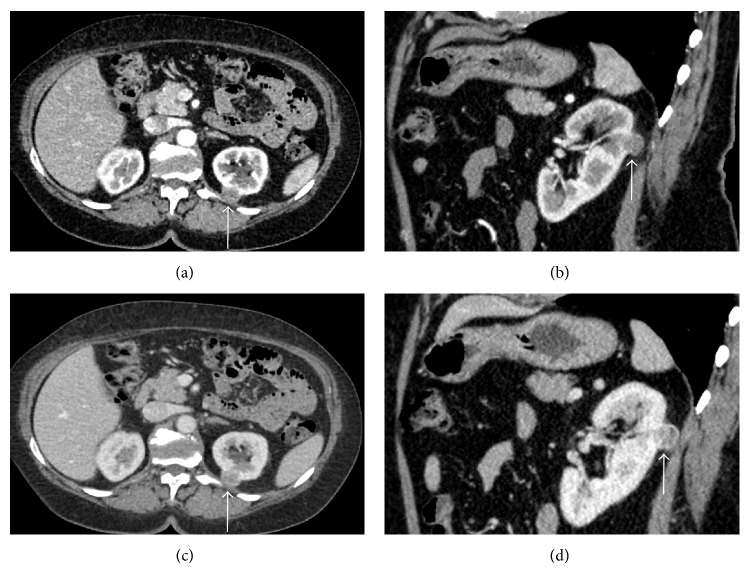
Oncocytoma. ((a)-(b)) Contrast-enhanced CT scans in arterial phase. ((c)-(d)) Portal phase CT scans. A small tumor with inhomogeneous aspect is recognizable at the anterior posterior portion of the left kidney (arrows).
